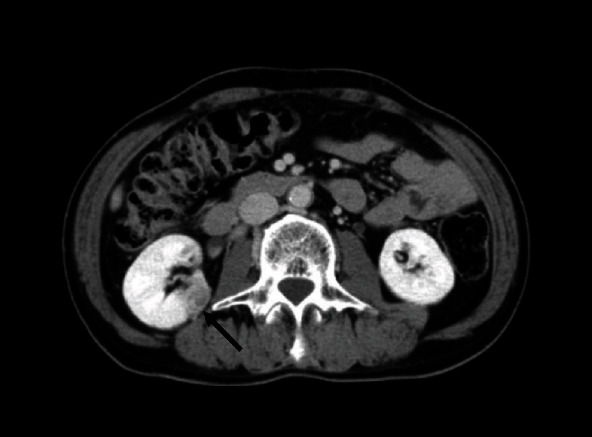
Contrast-enhanced computed tomography shows a right renal tumor (arrow) with a maximum diameter of 27 mm.
